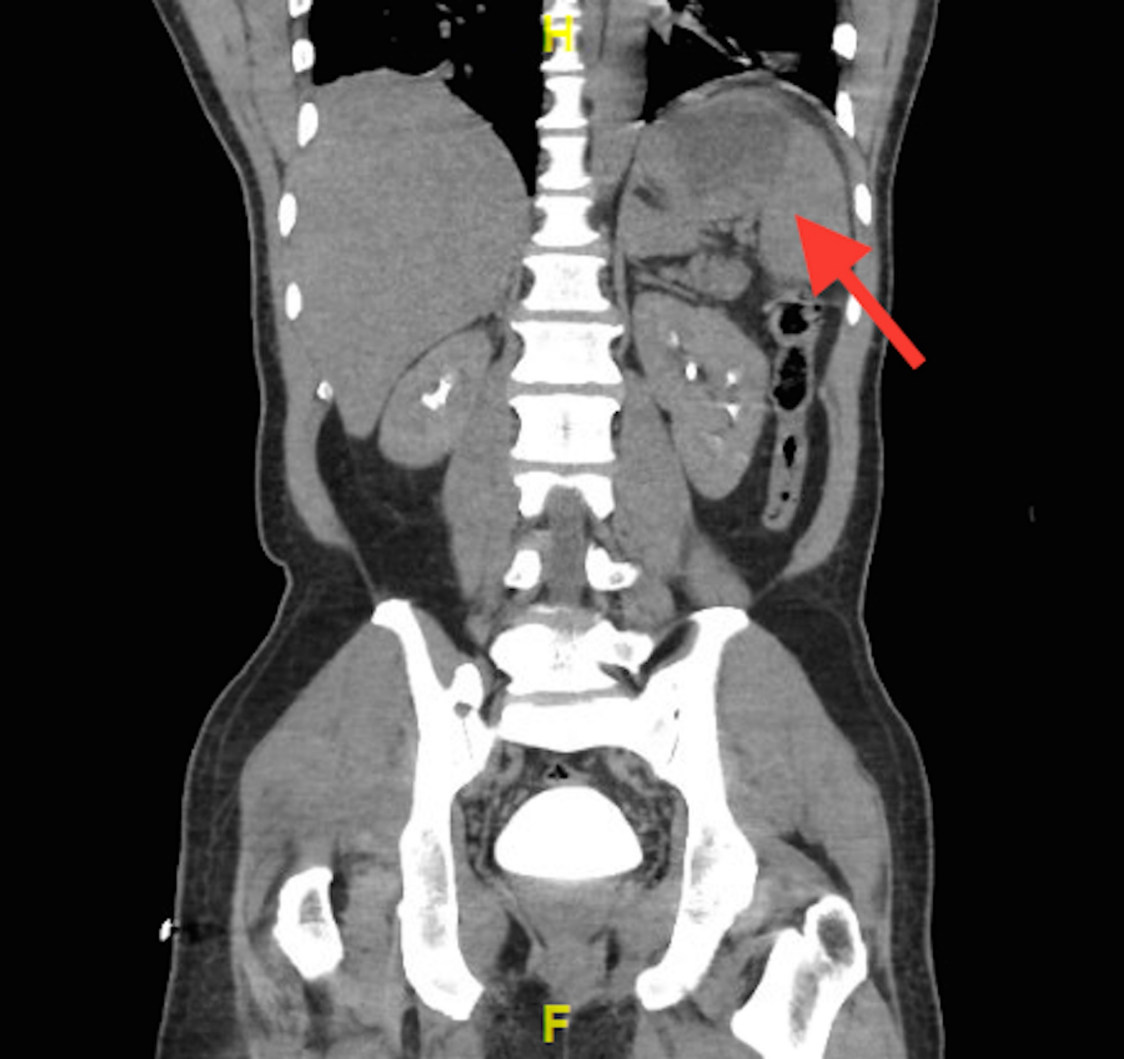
CT of the abdomen/pelvis coronal view demonstrating hypodensity in the spleen, read as infarct versus abscess
